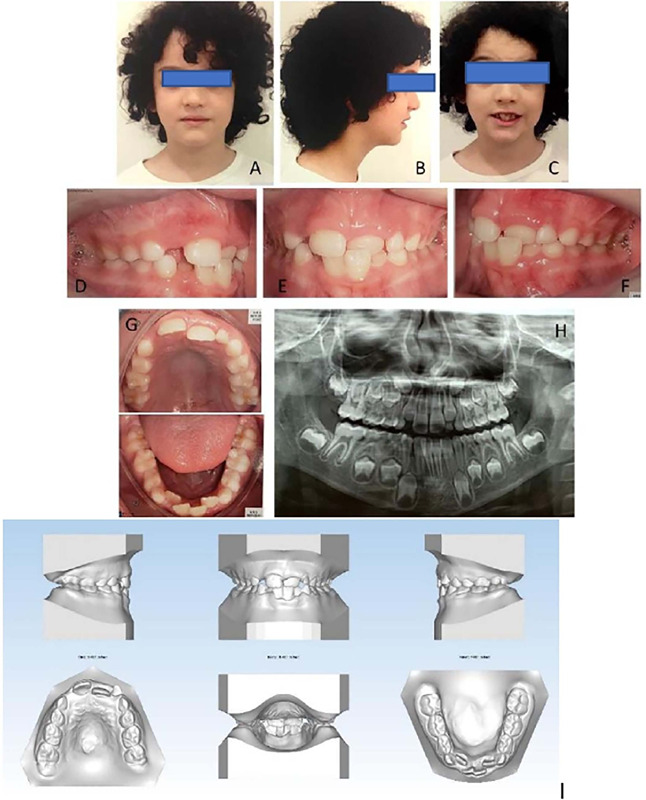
Pretreatment. (A-B-C) extraoral photographs; (D-E-F-G) intraoral photographs; (H) orthopantomograph (I) intraoral models.
